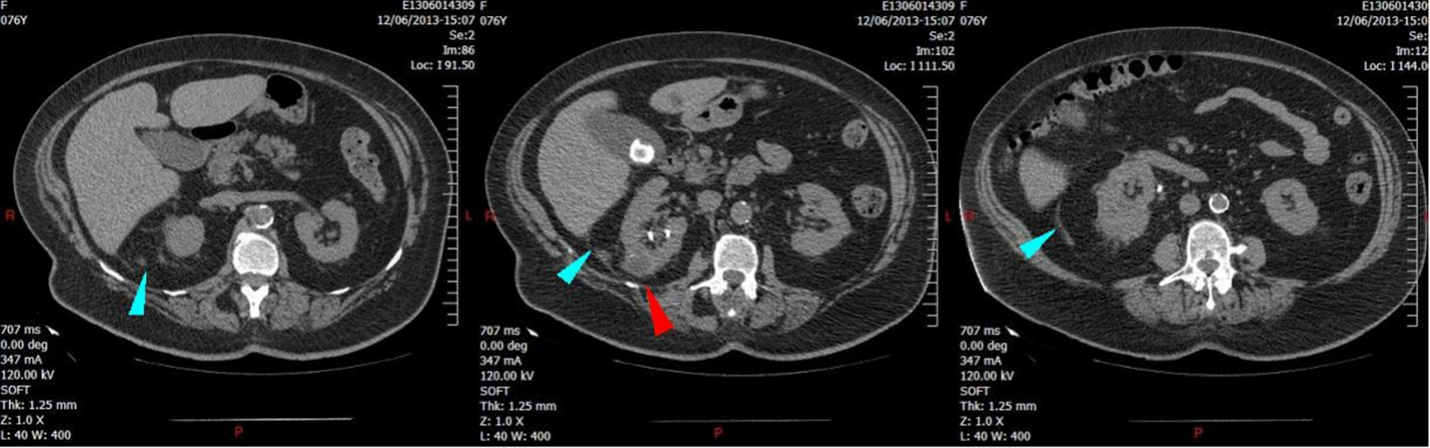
The 2-month follow-up computed tomography scan. The follow-up computed tomography scan shows the normalizing size and complete absence of gas in the right kidney. A small posterior cyst of the right kidney is still present (red arrow). Perirenal infiltration has almost disappeared (blue arrows).
